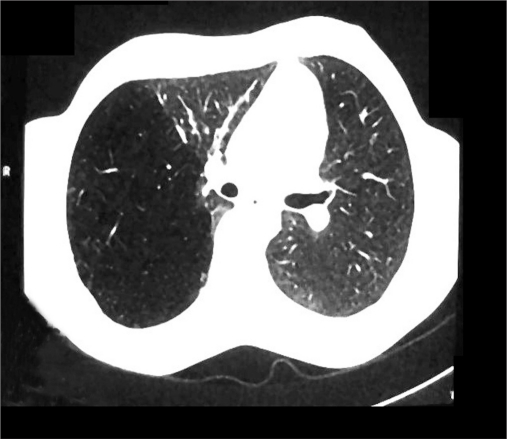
CT of chest showing hyperexpansion of posterior segment of right upper lobe and attenuated vascular markings
